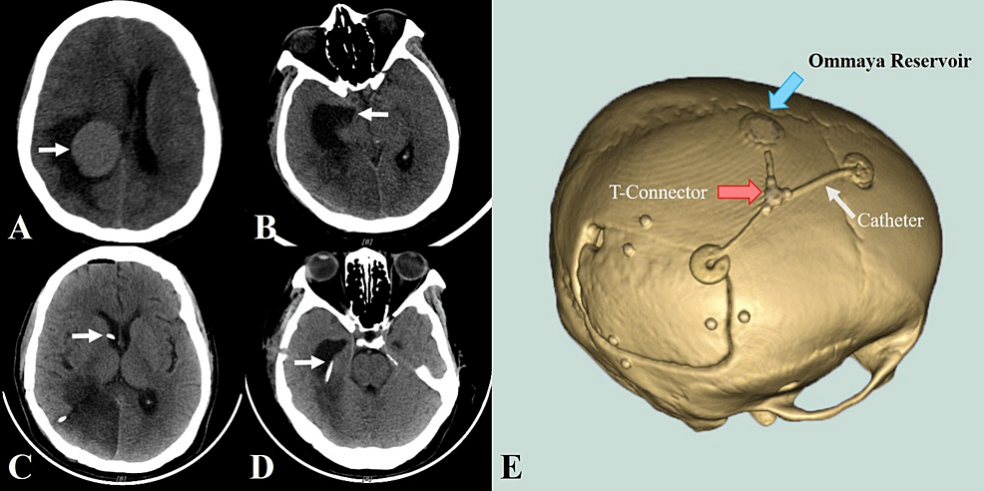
Image of case 1 A: CT scan showed a 4.2 cm × 3.4 cm meningioma in the right trigone with surrounding ventricular edema. B: CT scan demonstrated a temporal horn width of 3.87 cm. C: CT showed the catheter tip on the right frontal horn after the temporal-frontal horn shunt. D: CT showed the catheter tip on the right temporal horn after the temporal-frontal horn shunt. E: 3D reconstruction showed the position of the T-connector, Ommaya reservoir, and extracranial catheter.
